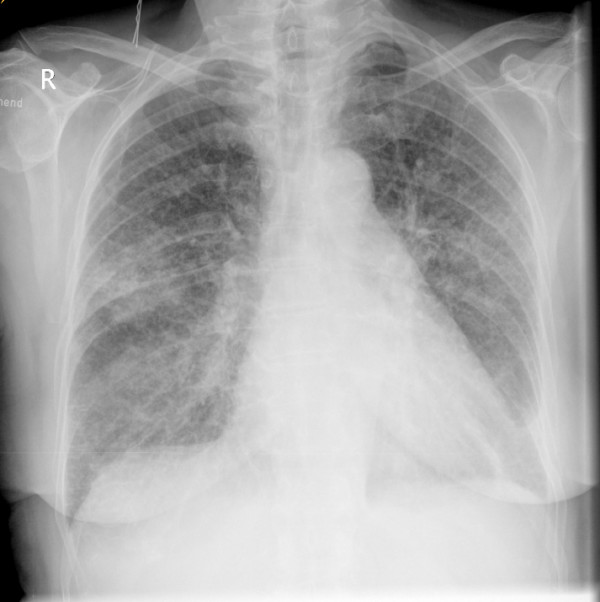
Thorax x-ray with diffuse small nodules and in some parts also confluent infiltrations; pleural effusion on the left side.
